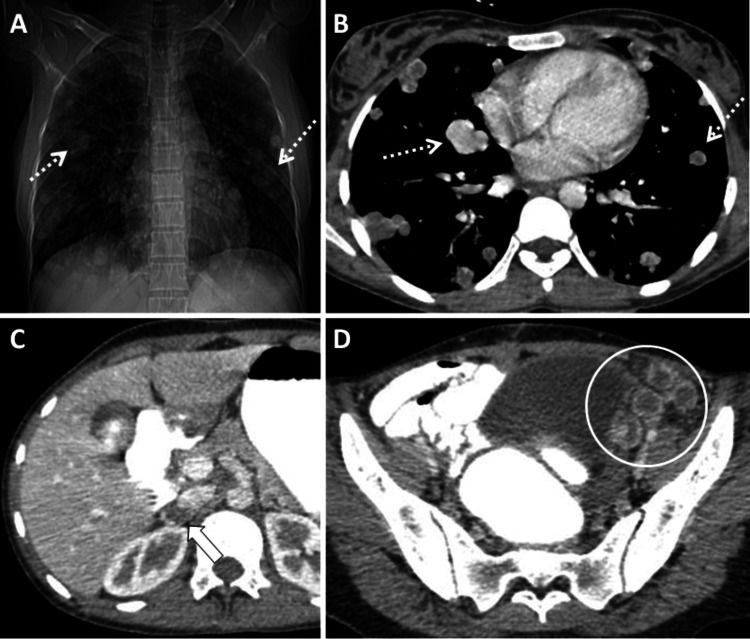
At two-month follow-up (A) chest radiography and (B) post-contrast CT chest show multiple lung parenchymal metastases with a typical cannonball appearance (dashed white arrows in A and B). (C and D) Post-contrast CT abdomen and pelvis demonstrate a right adrenal metastatic nodule (solid white arrow in C) and conglomerated necrotic metastatic deposits in the left lower quadrant along the external iliac chain (open white circle in C).
